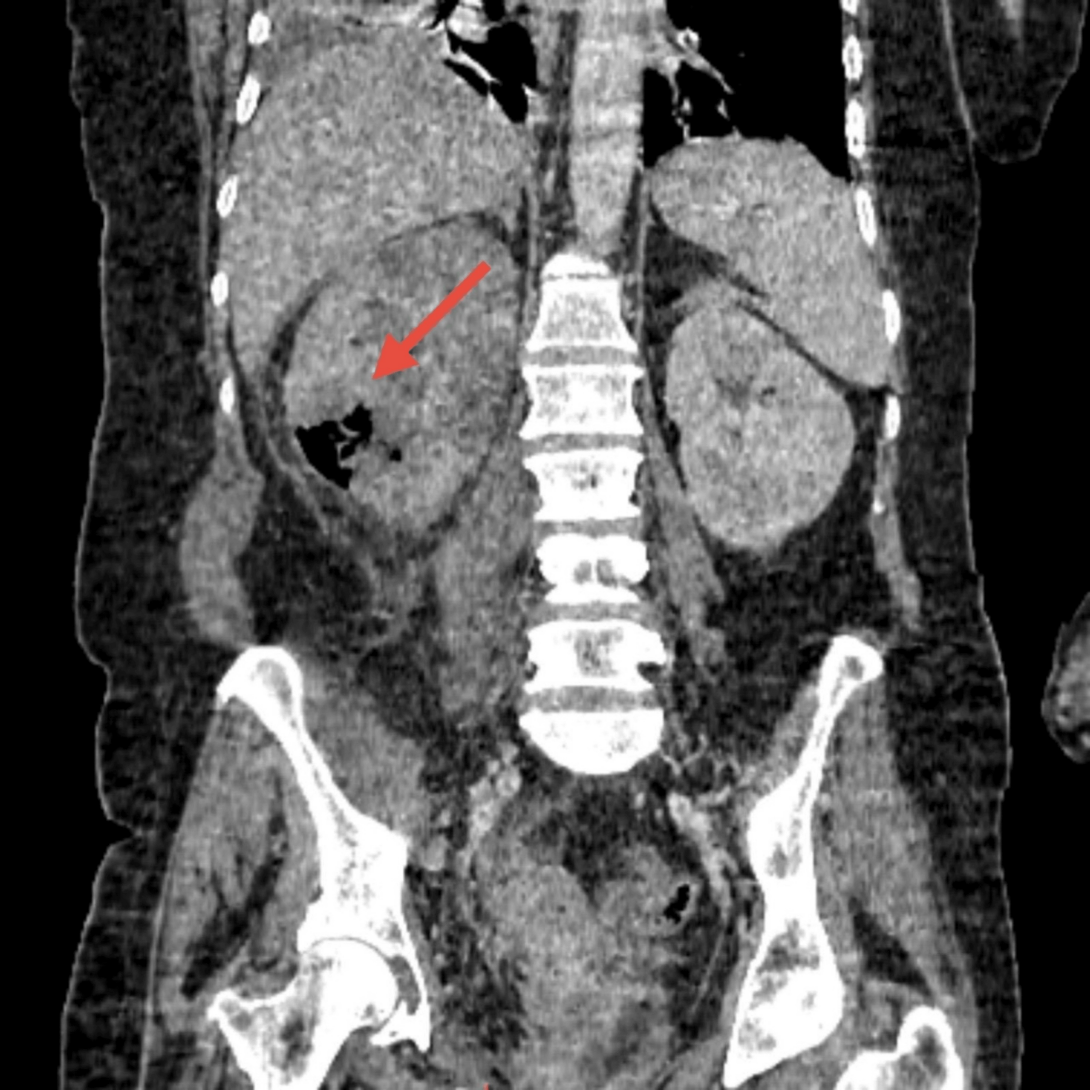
Coronal CT scan showing gas within the right renal parenchyma.
